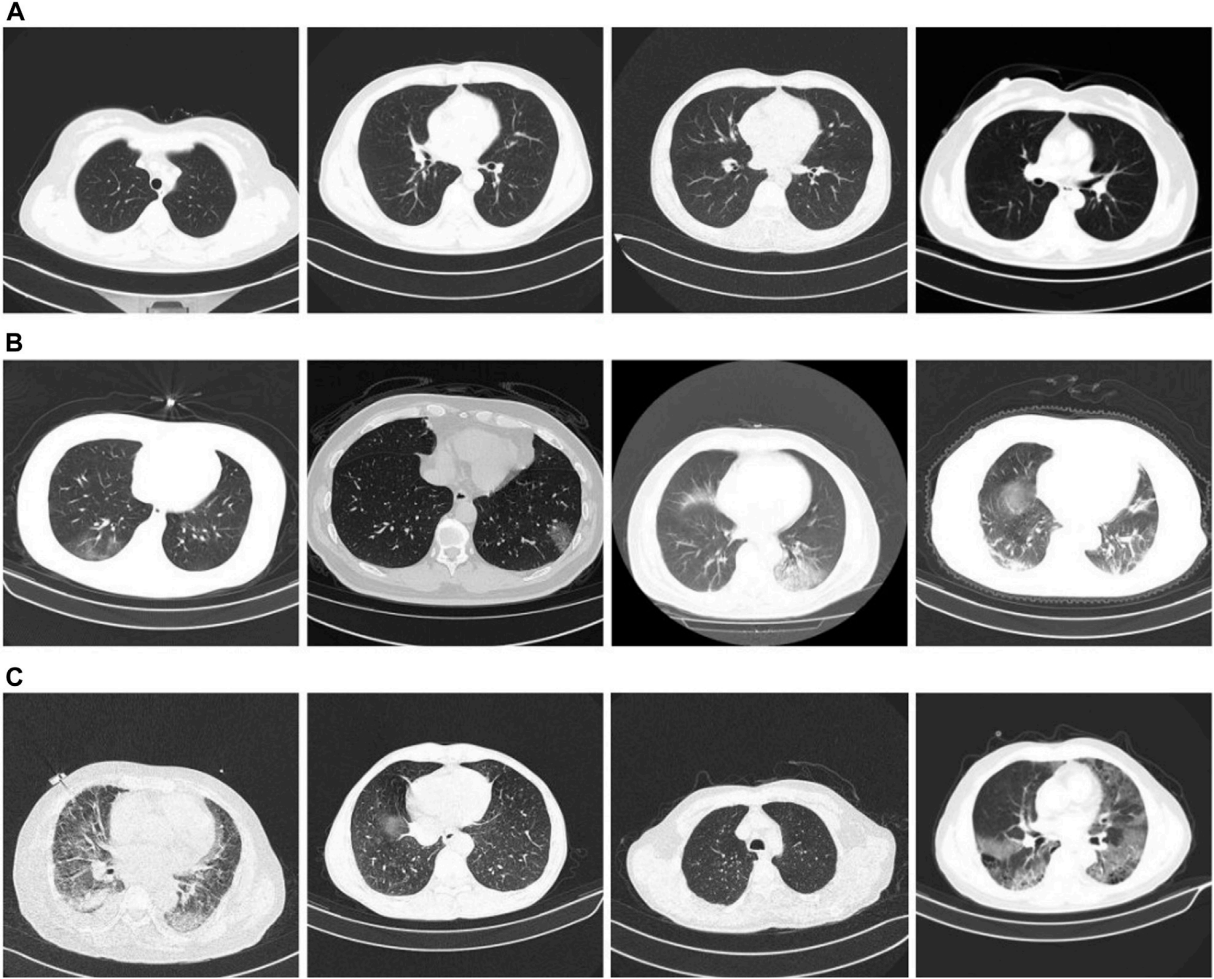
CC-CCII chest CT images. (A) Normal conditions; (B) COVID-19; (C) CP.
